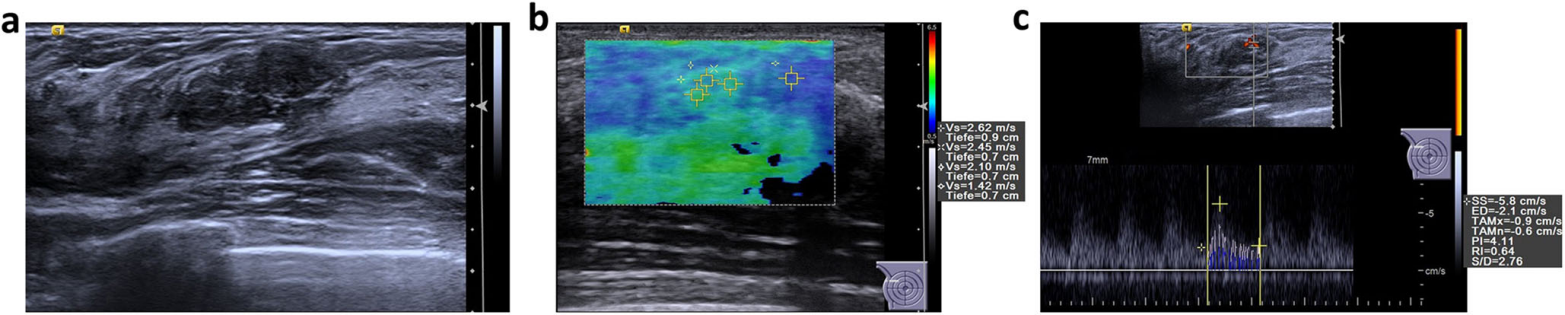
Multiparametric ultrasound of a palpable, 20-mm large lesion in the craniolateral right breast of a 23-year-old patient. a In B-mode, the lesion was heterogeneously hypoechoic, lobulated, with a partially indistinct margin and was classified as BI-RADS 4a. b In VTIQ elastography, the lesion was overall quite soft, with a SWVmax of 2.62 m/s. c Power Doppler detected internal vascularity and the spectrum analysis of the most prominent artery demonstrated an RI of 0.64. The application of the classification algorithm would classify this lesion as node 6, with probability of malignancy of 2.7 %, making a follow-up instead of biopsy a safe alternative. Ultrasound guided biopsy revealed an area of sclerosing adenosis
